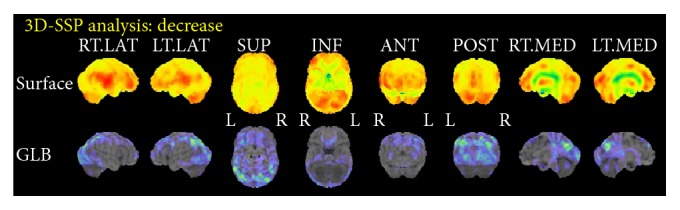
Three-dimensional stereotactic surface projections (3D-SSP) of single-photon emission computed tomography with N-isopropyl[^123^I]-p-iodoamphetamine (IMP-SPECT) of the patient in Case C. Decreased perfusion in the bilateral parietal cortex, precuneus, and posterior cingulate cortex indicated comorbid Alzheimer's disease.
